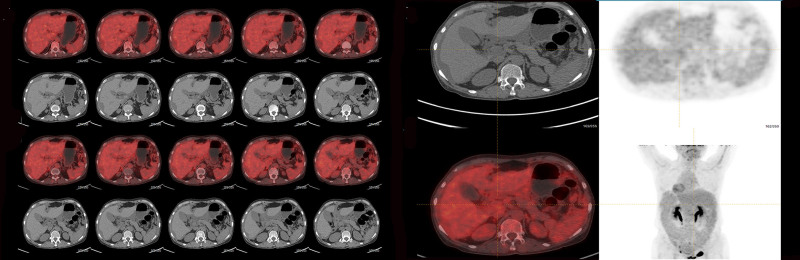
PET/CT scan showed irregular liver shape, widened portal vein, about 1.5 cm in diameter, and liquid density shadow was seen around the liver. PET/CT = positron emission tomography–computed tomography.
